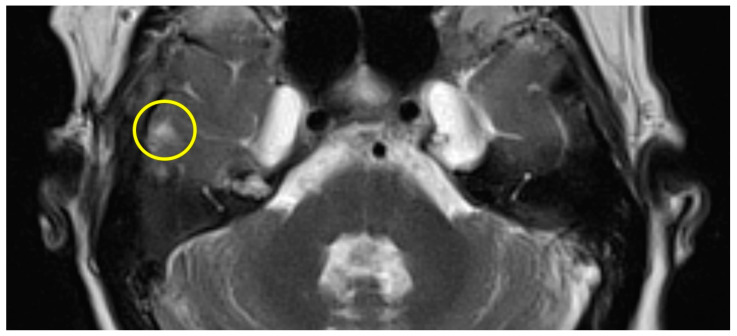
Axial T2w MR imaging of patient #2 (yellow circle = gliosis). Supplementary MR imaging can be downloaded.
